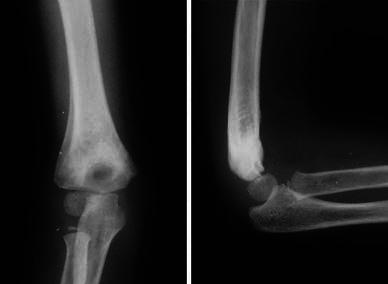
Fracture consolidation noted on the 3-month radiographs
